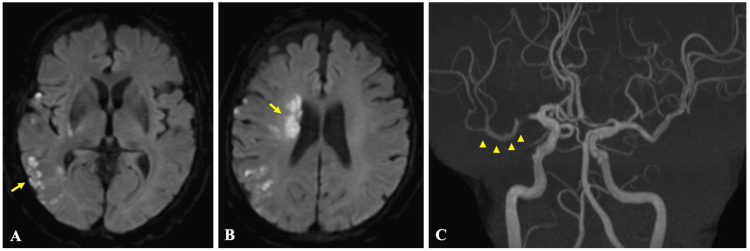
(A and B) Brain magnetic resonance imaging and (C) angiography at day 13 Axial diffusion-weighted images show new hyperintense lesions involving a portion of the right middle cerebral artery territory (A and B, arrow). Magnetic resonance angiography demonstrates that the M1 segment is nearly occluded with decreased distal flow signal beyond the stenotic segment (C, arrowhead)
